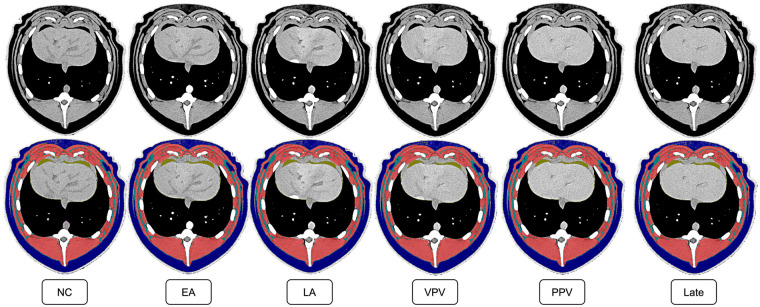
CT slices on the same level in the different CM phases, both without and with annotation of the BCA features. Axial CT slices on the thoracoabdominal junction with and without the segmentation of the BCA features in the different CM phases. The tissues were coded in color as follows; red: Muscle, dark blue: Subcutaneous adipose tissue, yellow: Visceral adipose tissue, light blue: Inter- and intramuscular adipose tissue. NC, non-contrast; EA, early arterial; LA, late arterial; VPV, vascular portal venous; PPV, parenchymal portal venous.
